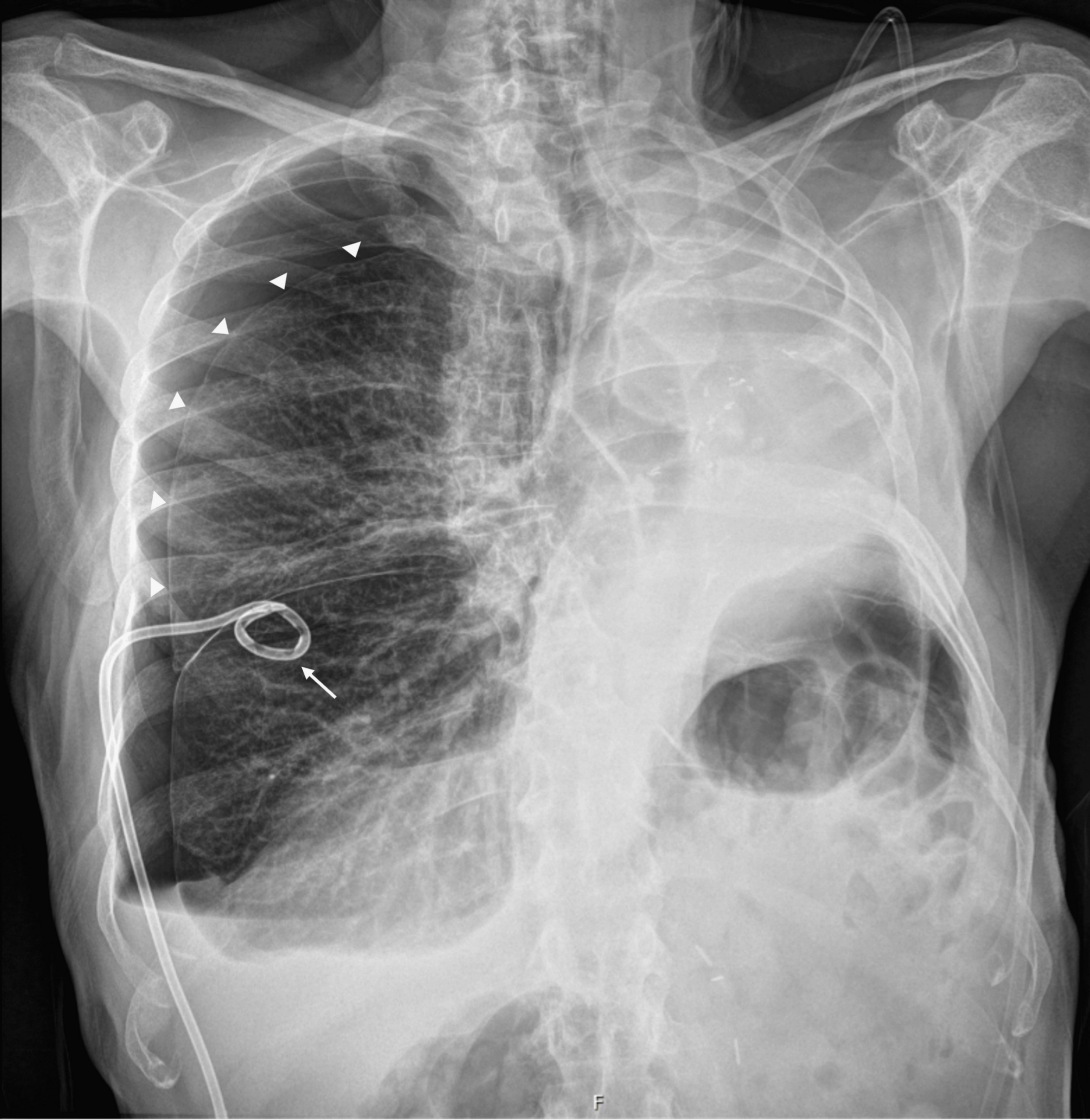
Chest radiography after chest tube insertion. Post-procedure chest radiograph demonstrating partial re-expansion of the right lung following the insertion of a 14-Fr pigtail chest tube. Arrowheads indicate the visceral pleural line, consistent with a residual pneumothorax, and the arrow denotes the pleural drainage catheter. The changes observed on the left side are consistent with a prior pneumonectomy.
